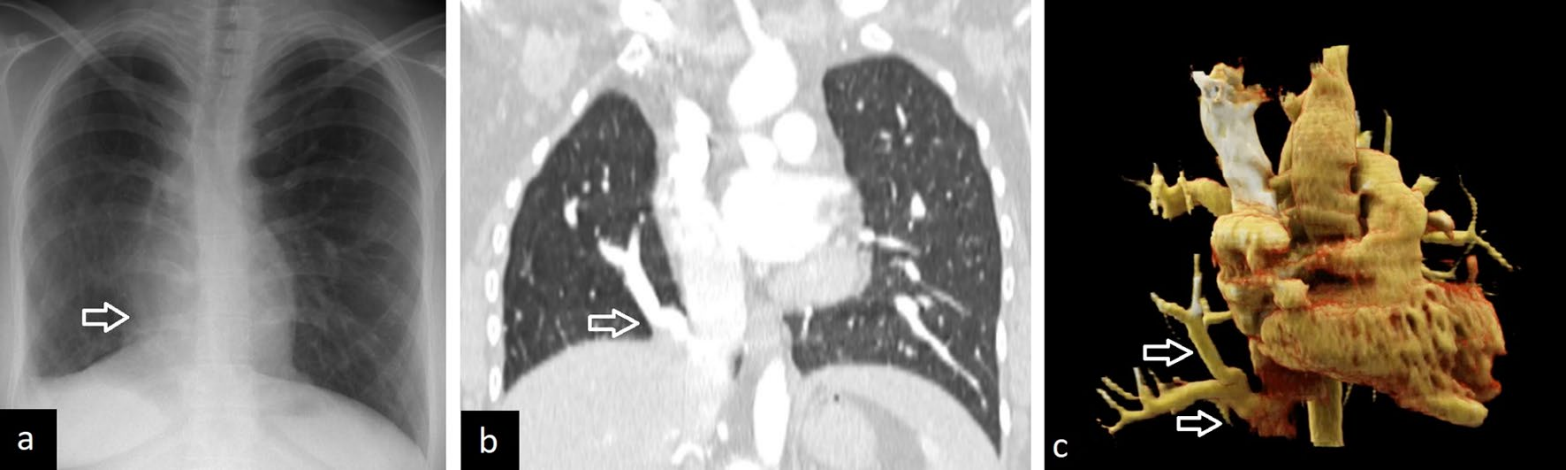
A 25-year-old female with scimitar syndrome. Chest X-ray (a) and coronal CT image (b) demonstrate right lung hypoplasia and curvilinear tubular opacity joining the inferior vena cava above the medial aspect of the right hemidiaphragm (arrows). 3D reconstruction image obtained with the cinematic volume-rendering-technique shows (c) the way of the anomalous pulmonary vein (arrows)
